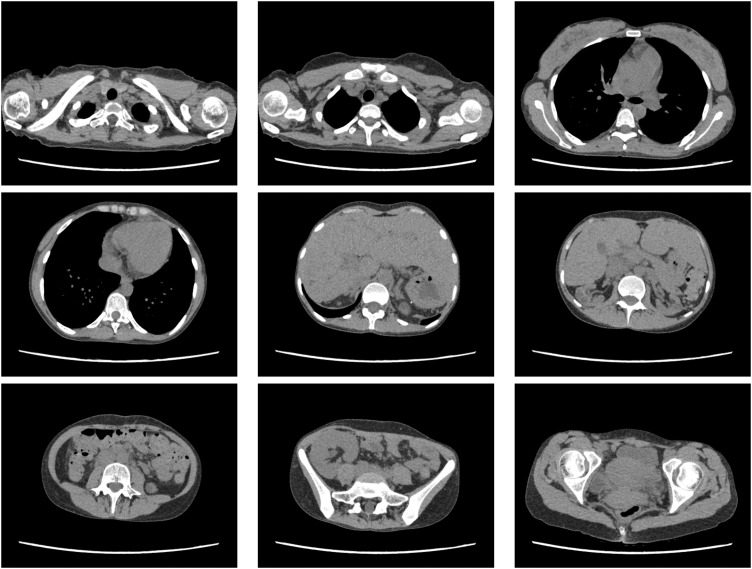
Chest and abdominal CT images showed no mass lesions other than in the transplanted kidney. No neoplastic lesions were identified other than the transplanted kidney.
